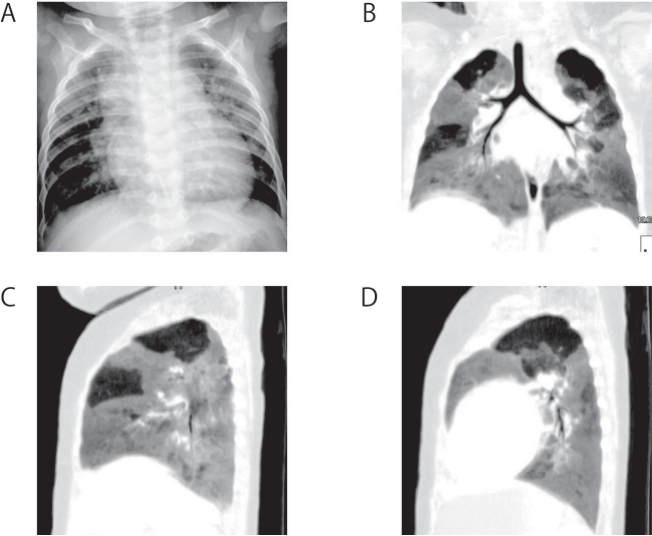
A 6-month-old girl with ventricular septal defect. Emphysematous change is not recognised with chest X-ray (A). Coronal view (B), sagittal view of right lung (C), and sagittal view of left lung (D) minimum-intensity projection imagings show that there are segmental emphysema in S1, 4 of right lung, and S1-2, 6, 7-8 of left lung. *Reproduced with permission from Heart, Lung and Circulation 2011, Elsevier limitied.*
